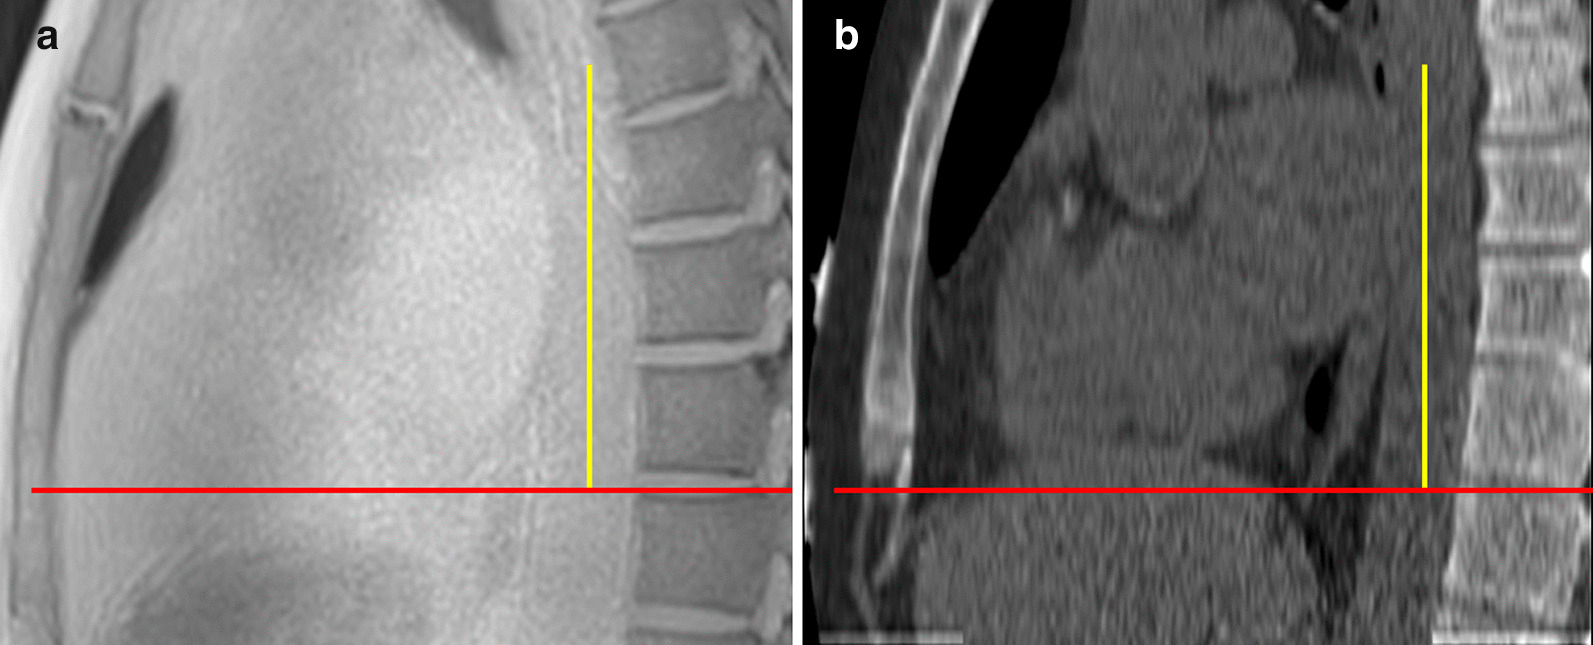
Sagittal (a) radial-volumetric interpolated breath-hold examination (VIBE) cardiovascular magnetic resonance (CMR) and (b) computed-tomography (CT) images of descending thoracic aorta. A 9 cm segment of thoracic aorta from the same patient is chosen on both CT and radial-VIBE images. Red horizontal lines mark the level of the top of the vertebra that is closest to the inferior surface of the heart. Yellow vertical lines correspond to 9 cm of thoracic aorta
